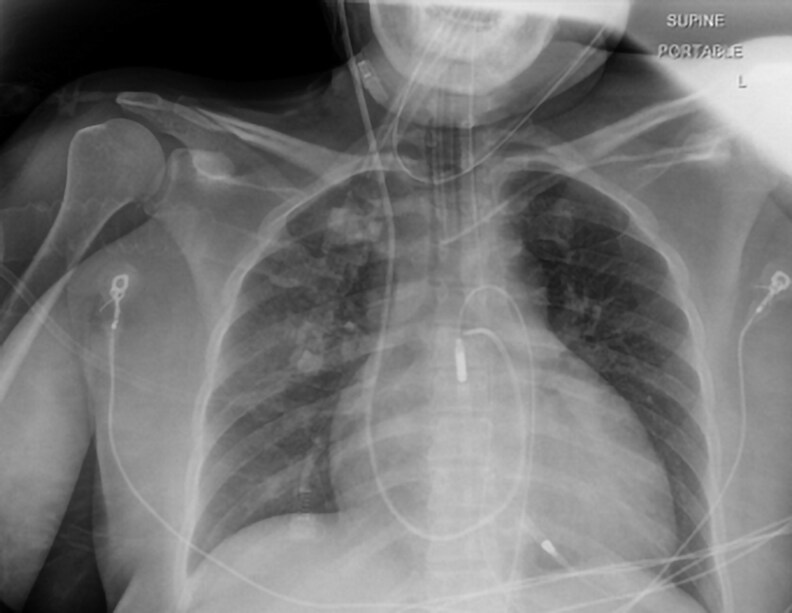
Initial chest radiograph demonstrating cardiomegaly and pulmonary vascular congestion.
